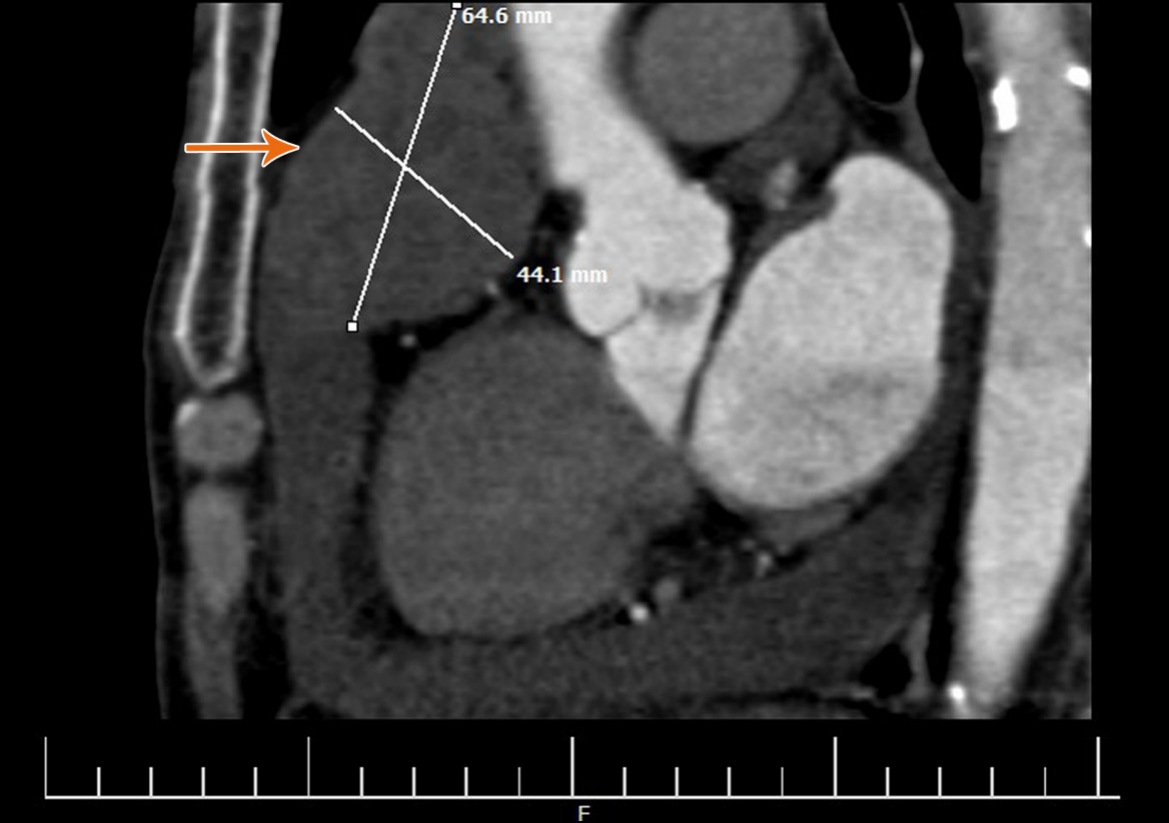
Coronal view of the mediastinal mass (orange arrow) in multislice spiral thoracic computed tomography scan with contrast
